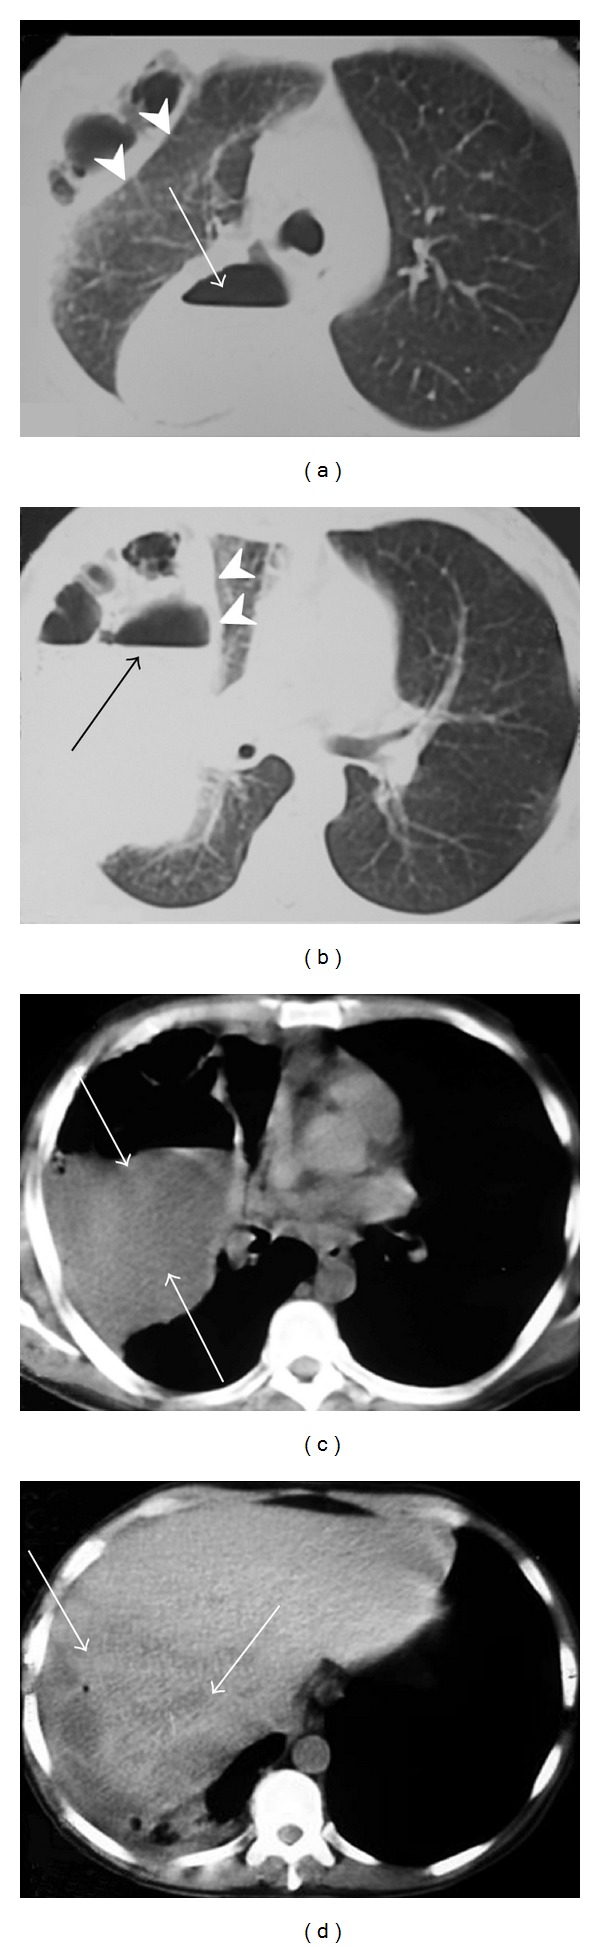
(a) and (b) computed tomography showing a collapsed right lung (arrow heads) and gas-fluid levels in the right hemithorax (arrows); (c) pleural effusion (arrows); (d) hepatic mass in segments VI and VII (arrows).
